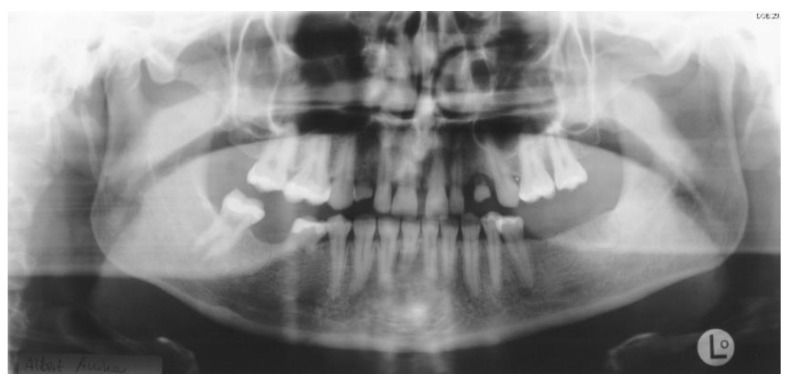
Initial panoramic radiography.
